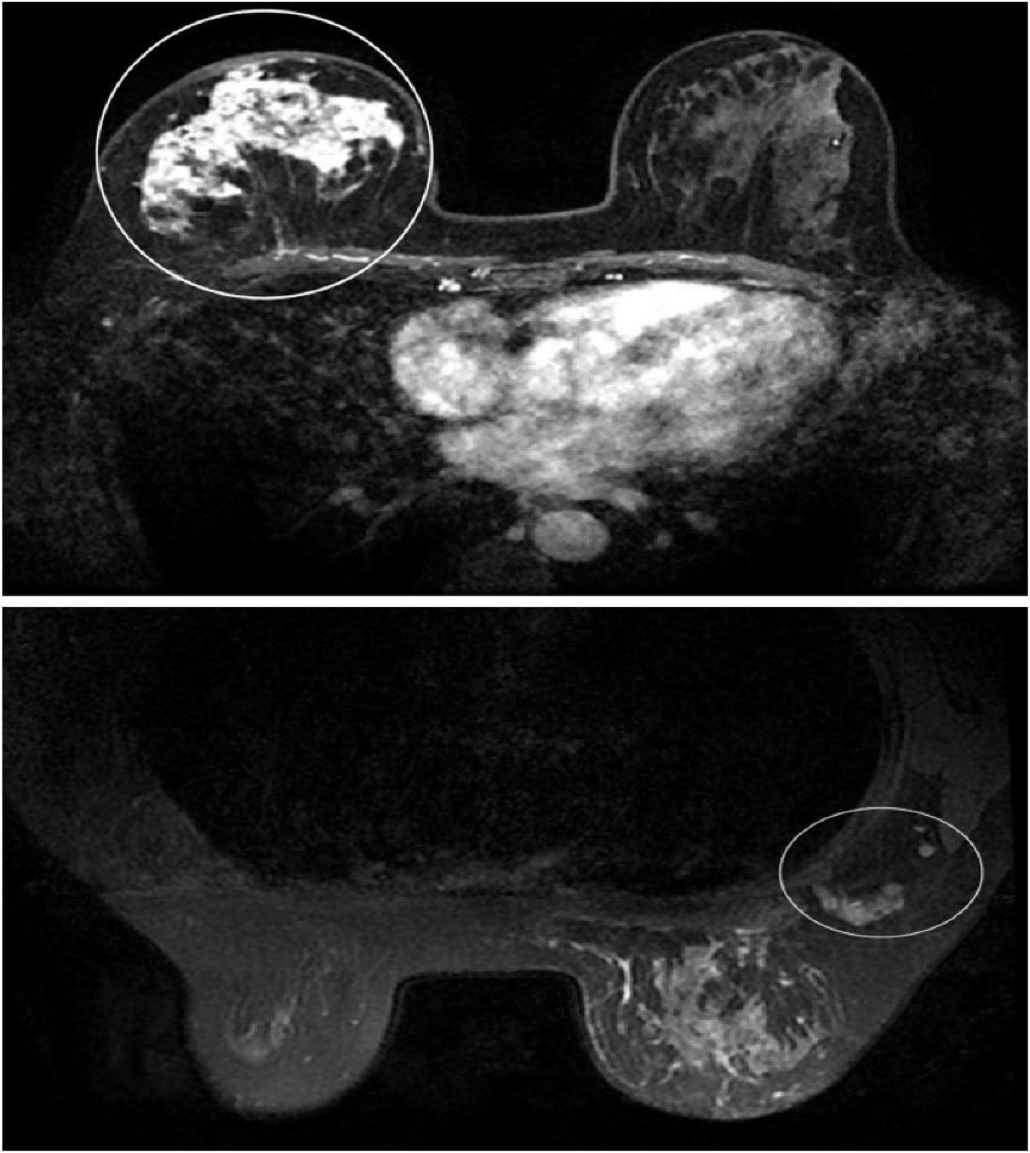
The baseline breast MRI revealed non-mass-like enhancement in the right breast, measuring 7.5× 5.2 × 8.4 cm. Multiple enlarged lymph nodes are visible in the right axilla, with the largest measuring approximately 1.5 cm in short diameter.
